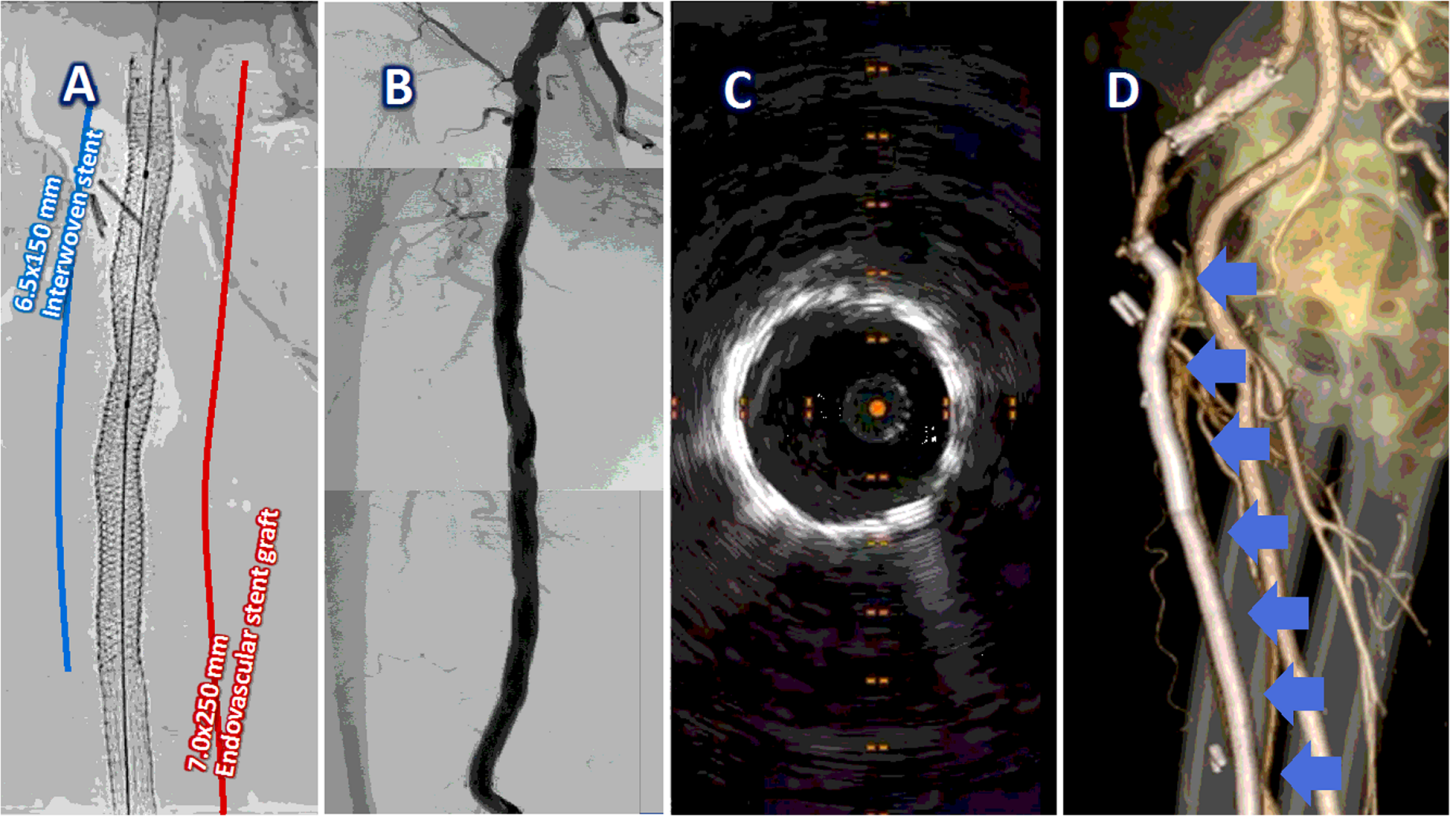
Final results and computed tomography angiogram at the 12-month follow-up. A. The lesion was fully covered with a 7.0 × 250-mm endovascular stent graft (red line), and a 6.5 × 150-mm interwoven stent (blue line) was implanted to resist recoil and extrinsic compression. B. Final angiogram demonstrates a restored blood flow. C. Intravascular ultrasound showed symmetrically expanded scaffolds. D. Computed tomography angiogram found patency (blue arrows) one year after the treatment
